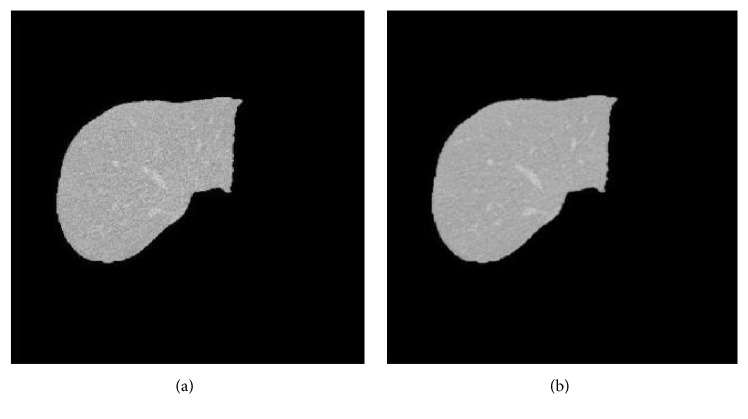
Preprocessing image on the original CT scan images. (a) Image before applying the filter and (b) image after applying the filter (preprocessing).
